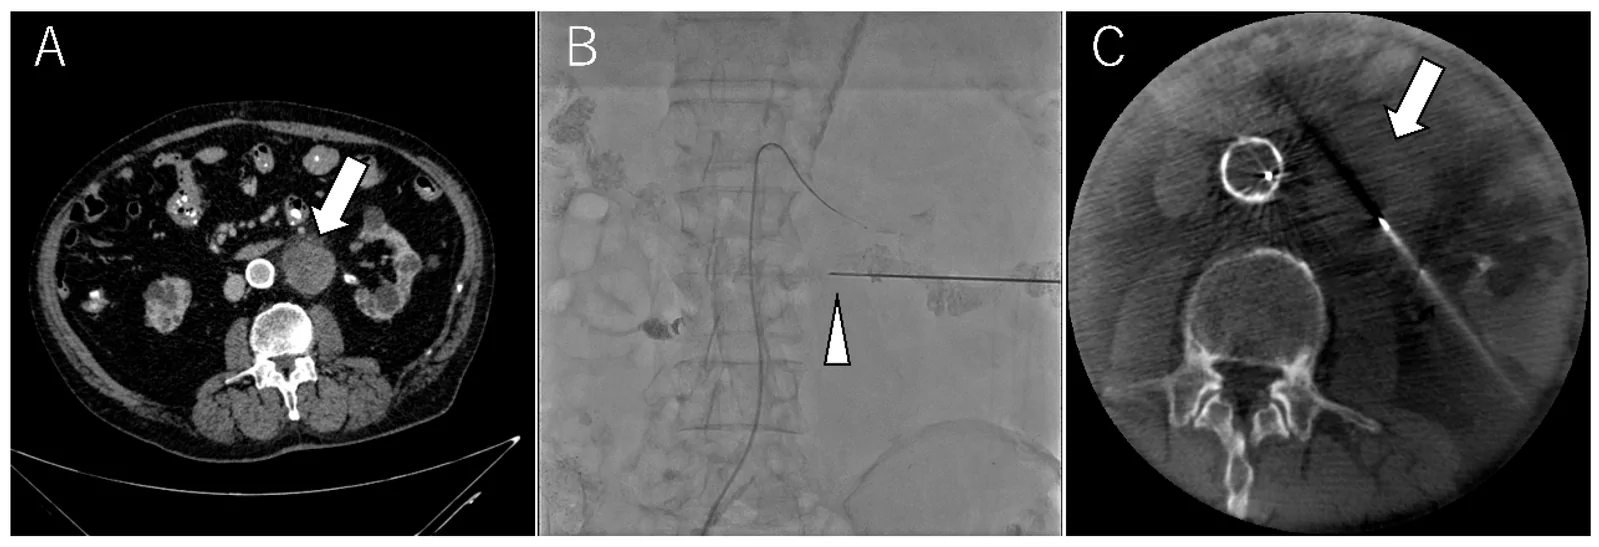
Cone-beam CT–guided biopsy of a retroperitoneal mass using a dorsal-space mat with a posterior approach. (A) Preprocedural contrast-enhanced CT image. A mass with surrounding inflammatory changes (arrow) is seen on the left side of the infrarenal abdominal aorta, raising suspicion for an infected thrombosed aneurysm. (B) Fluoroscopic spot image during puncture. Because biopsy was required to exclude malignancy, the procedure was performed in the angiography suite to allow rapid embolization if needed. Cone-beam CT–guided biopsy was performed using a posterior approach while the patient remained supine (arrowhead: biopsy needle). (C) Cone-beam CT image to confirm needle position. The biopsy needle reaches the mass (arrow) via a posterior approach. After advancing the needle an additional 1 cm, tissue sampling was performed under fluoroscopy.
